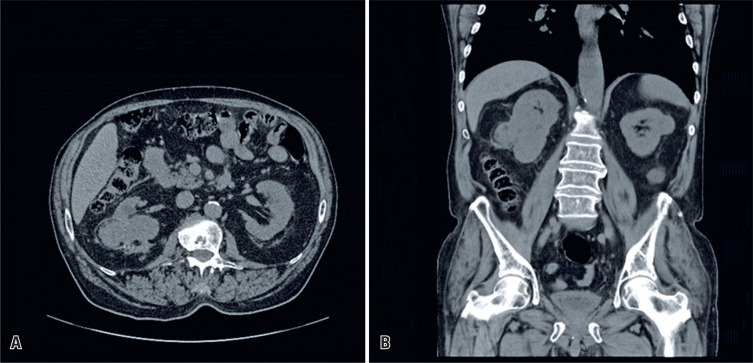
Axial (A) and coronal (B) non-enhanced computed tomography images (Soft tissues window) showing the size reduction in the angiomyolipomas after 3 months
